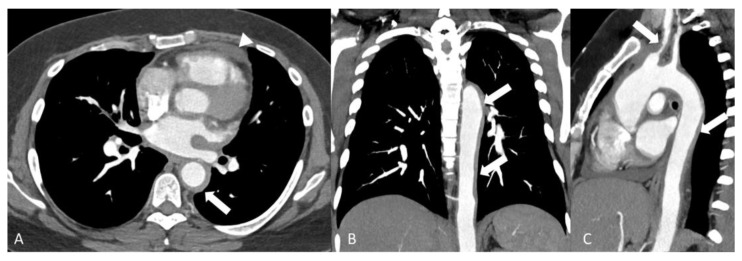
A 28-year-old man with active Takayasu’s disease. Axial (A), coronal (B) and sagittal (C) images of chest CTA demonstrating concentric mural thickening involving the ascending aorta, arch, descending aorta and proximal right brachiocephalic artery (arrows) and small reactive pericardial effusion (arrowhead).
